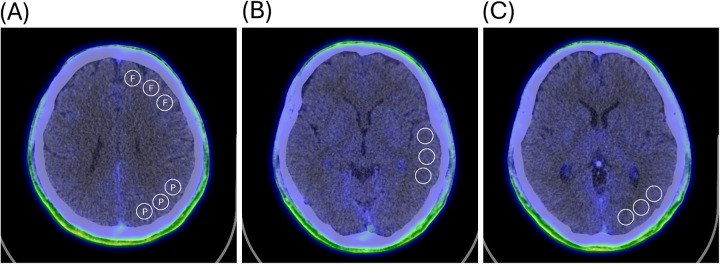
Manual placement of VOIs in each area of the head. Representative VOI placement in contralateral normal-appearing cerebral cortex. Three 1.5-cm spherical VOIs were placed in gray matter corresponding to each lesion for mean SUV measurement. (A) Frontal and parietal lobes (F, frontal; P, parietal); (B) temporal lobe; (C) occipital lobe.
